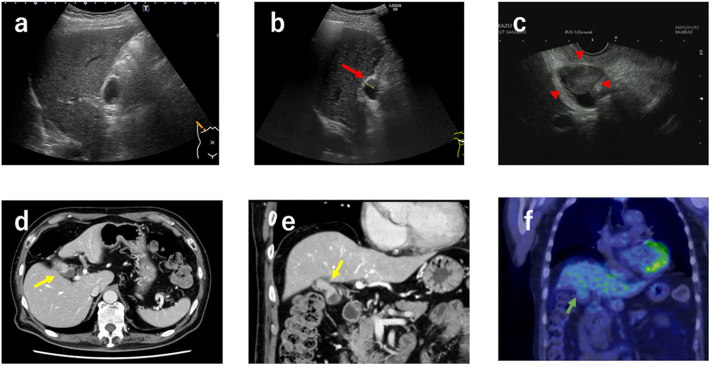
Preoperative transabdominal ultrasonography(a–b), endoscopic ultrasonography (EUS) (c), computed tomography (CT) (d), EUS (e), and positron emission tomography (PET)-CT(f). (a) Previous year's transabdominal ultrasonography finding was normal. (b) In this US, wall thickening is noted (red arrow). (c) Endoscopic ultrasonography reveals subpedunculated, broad-based, elevated lesions with irregular surfaces (red arrow head). (d–e) A tumor is found that protruded into the lumen of the bottom of the gallbladder in the contrast-enhanced (yellow arrow) CT. (f) PET-CT shows mild uptake of fluorodeoxyglucose (FDG) in the tumor (green arrow). (For interpretation of the references to colour in this figure legend, the reader is referred to the web version of this article.)
